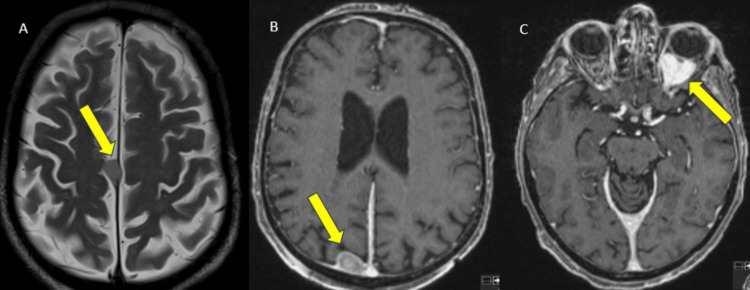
Axial T2-weighted imaging (WI) (A) and T1 fat-saturated post-gadolinium (T1FS Gd) (B and C) MRI of the brain depicts extra-axial dural-based lesions attached to the mid one-third of the falx cerebri (A, yellow arrow) and another in the right posterior parasagittal parietal convexity (B, yellow arrow). Left intraorbital, intraconal lesion is seen in C (yellow arrow). The lesions demonstrated avid enhancement (seen in B and C) MRI: magnetic resonance imaging
